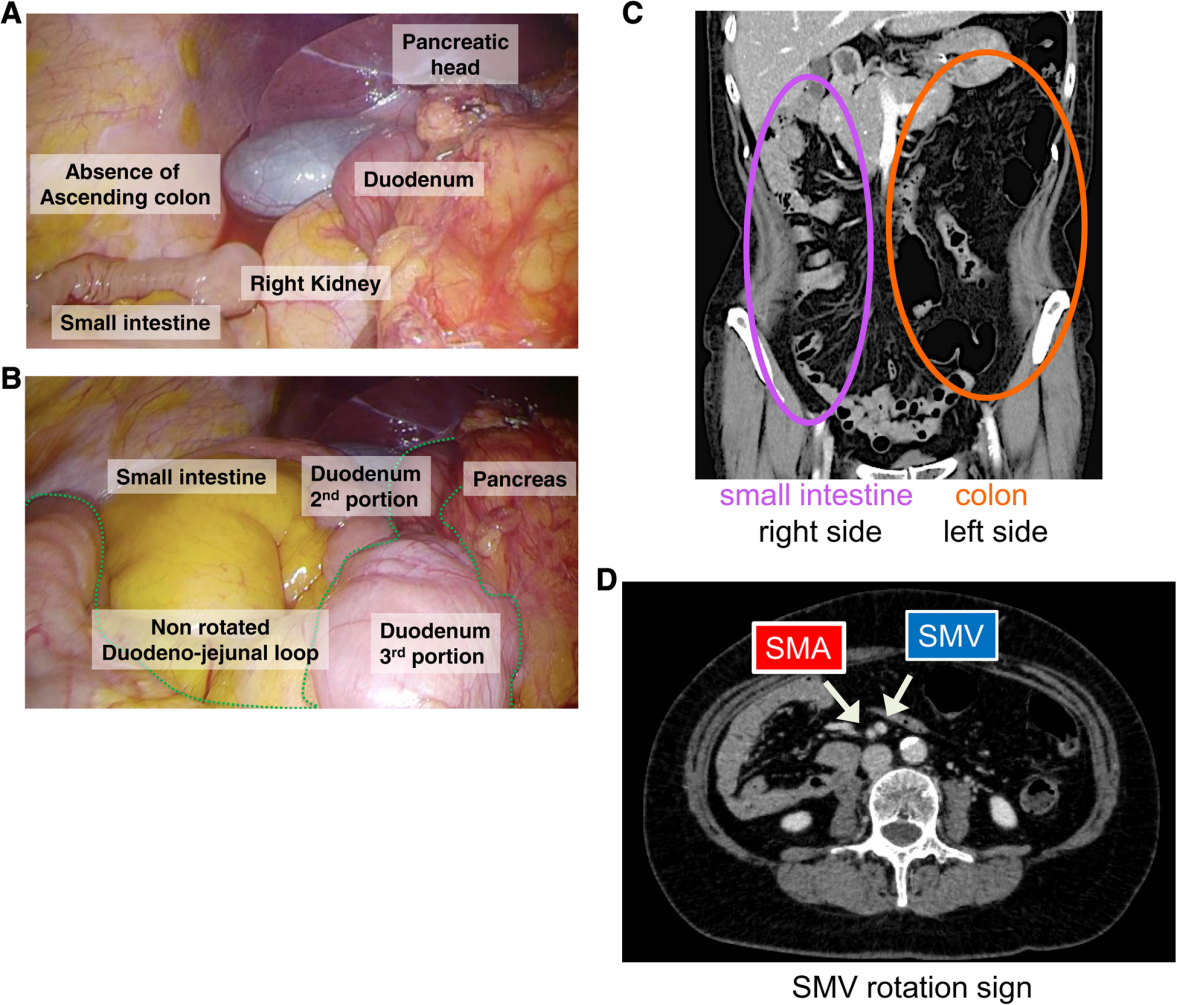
Operative views before reconstruction and CT findings, indicating asymptomatic intestinal malrotation. a,b There was no right-side colon at the hepatic flexure, and the duodenum did not rotate to the left side of the body. The small intestine was located on the right side of the abdomen, with a completely mobilized duodenum. c A right-sided small bowel and left-sided colon sign were confirmed on reviewing the CT image. d SMV rotation sign: SMV existed abnormally on the left side of the superior mesenteric artery (SMA)
